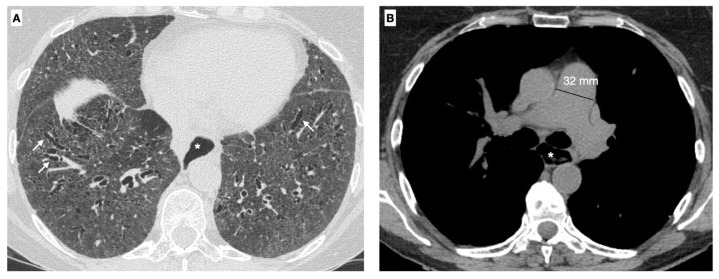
A 63-year-old female with the diagnosis of SSc. (A) Axial High-resolution CT scan shows traction bronchiectasis (white arrows) in a back-ground of diffuse ground-glass opacities, related to a fibrotic nonspecific pneumonia (NSIP) patter. (B) Using the mediastinal window setting the dilatation of the main pulmonary artery (32 mm) can also be recognized as sign of pulmonary hypertension, a common complication of the disease. Moreover a dilatation of the esophagus (*) can be seen (operator E.B., Radiology Unit, University of Trieste, University Hospital of Cattinara).
